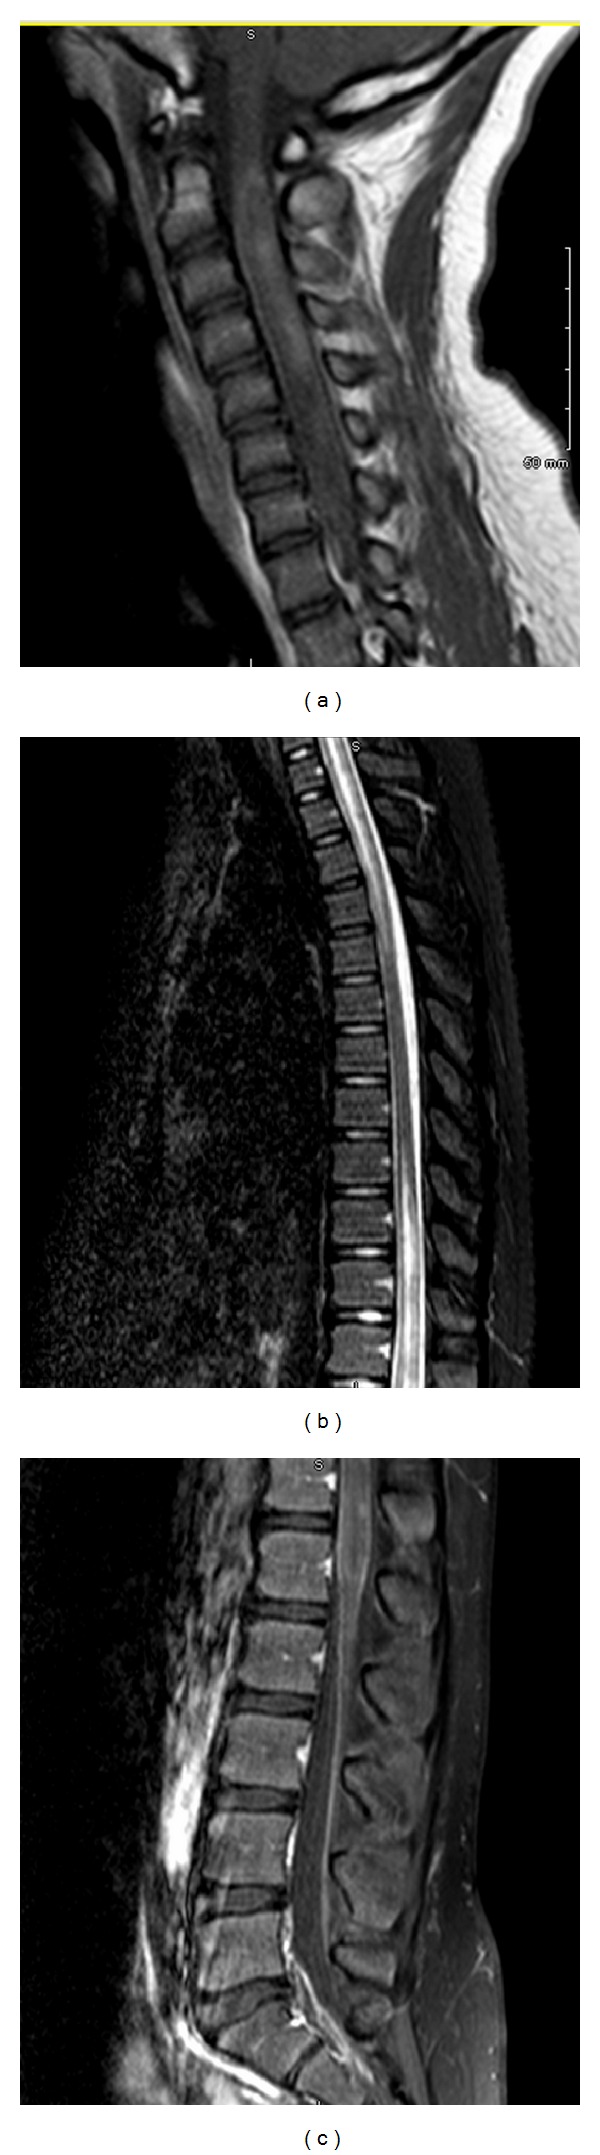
MRI of the cervical spine: (a) T1W postcontrast shows increased cord signal with an enlarged, edematous central cord. MRI of the thoracic spine: (b) T2W STIR shows abnormal T2 signal within the cord. MRI of the lumbar spine: (c) T1W postcontrast with fat suppression shows abnormal enhancement in the cord.
